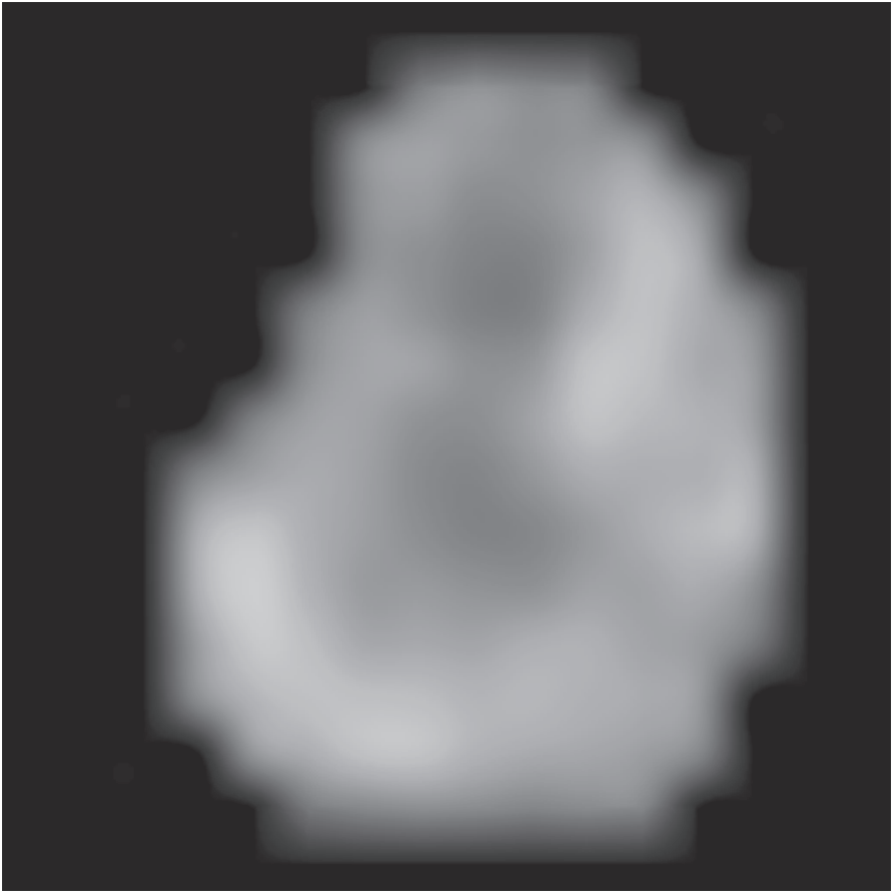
Variations in the grayscale intensity of this tumor are evident. For example, contrast the bright region at the bottom-left to the darker region in the center. The regions of varying intensity are amorphous an diffusely bounded. In the present case, these regions represent the heterogeneous uptake of a radio-labeled glucose analogue (FDG). The vertical edge of this image corresponds to 64 mm. This image is also seen in Figure
